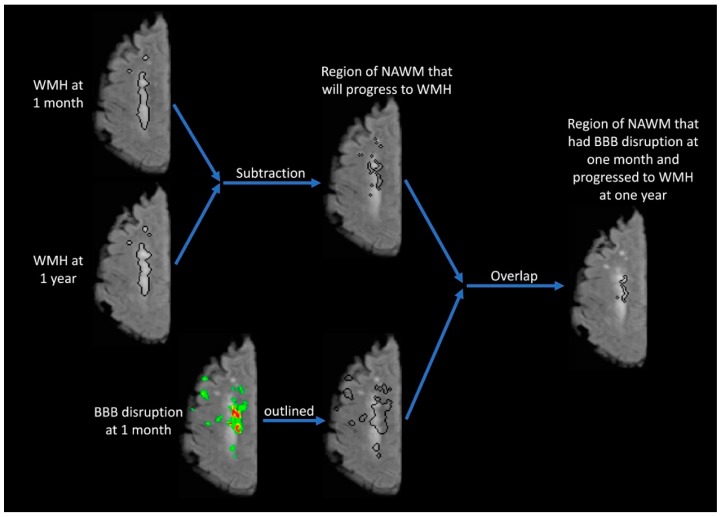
The purpose of this figure is to identify overlap between the location of BBB disruption 1 month after stroke and the locations of new white matter hyperintensities (WMH) at 1 year after stroke. The WMH region of interest (ROI) from 1 month is subtracted from the ROI at 1 year to identify regions of new WMH that are then superimposed on the 1-month FLAIR. Thus, the new ROI is an area of normal appearing white matter (NAWM) from the 1-month time point that will progress to WMH at the 1-year time point. Below that, the BBB disruption from the 1-month time point is outlined to form an ROI. The overlap between the BBB ROI and the ROI of new WMH is then identified to create a final ROI that identifies WHM progression that was preceded by BBB disruption.
